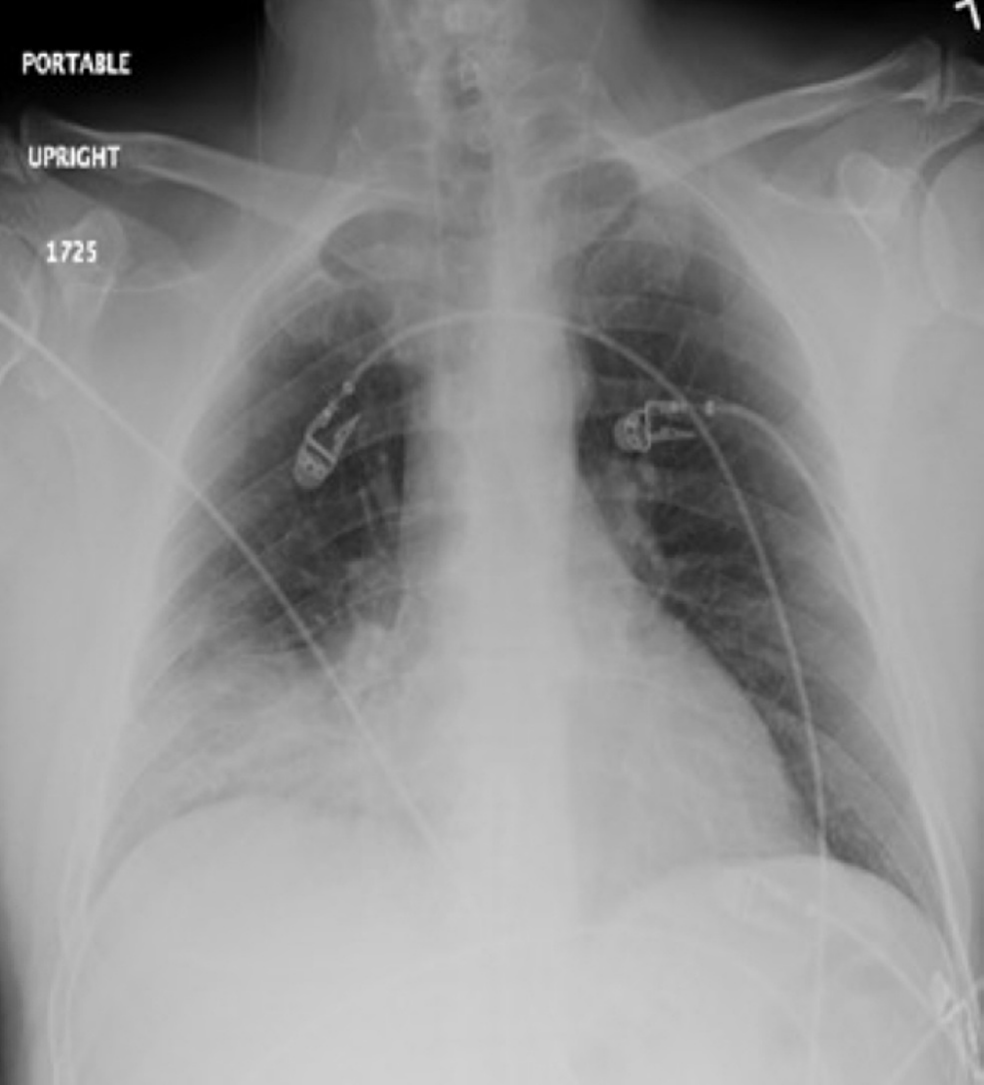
Chest radiograph demonstrating right middle and lower lobe infiltrates.
